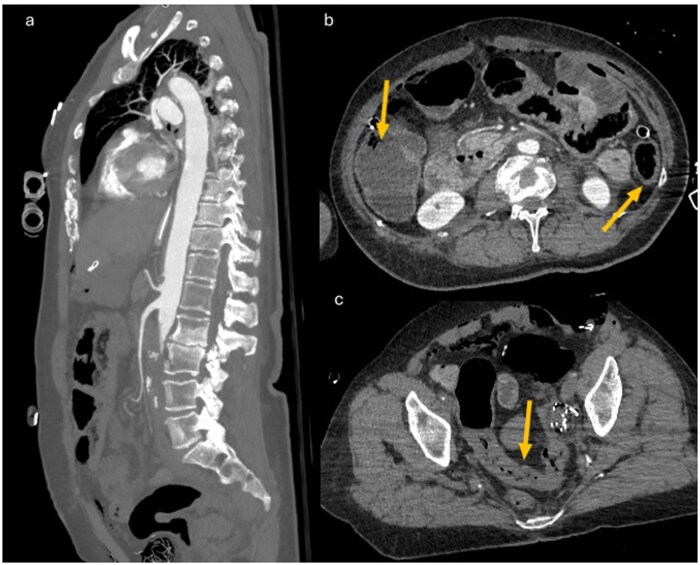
Multiplanar and axial CT findings of intestinal ischemia secondary to acute inferior mesenteric artery occlusion. (a) Sagittal MPR reconstruction in the arterial phase shows normal opacification of the superior mesenteric artery (SMA), while the inferior mesenteric artery (IMA) is not visualized, its origin being located within the segment of thrombosed abdominal aorta. Axial venous-phase images demonstrate preserved enhancement of the ascending colon, supplied by the SMA (arrows in b), contrasted with hypoenhancement and mural thickening of the descending and sigmoid colon, consistent with intestinal ischemia in the IMA territory (arrows in c). Signs of postoperative pneumoperitoneum are also visible, related to the recent abdominal surgery.
